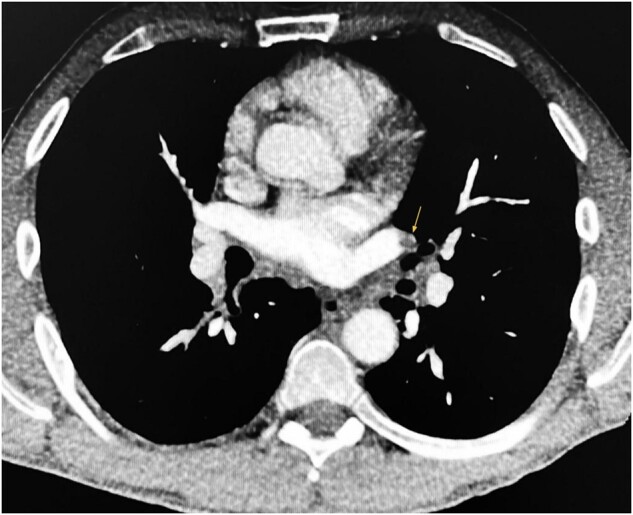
Contrast-enhanced chest CT scan showing pulmonary embolism in the left pulmonary artery (arrow).
